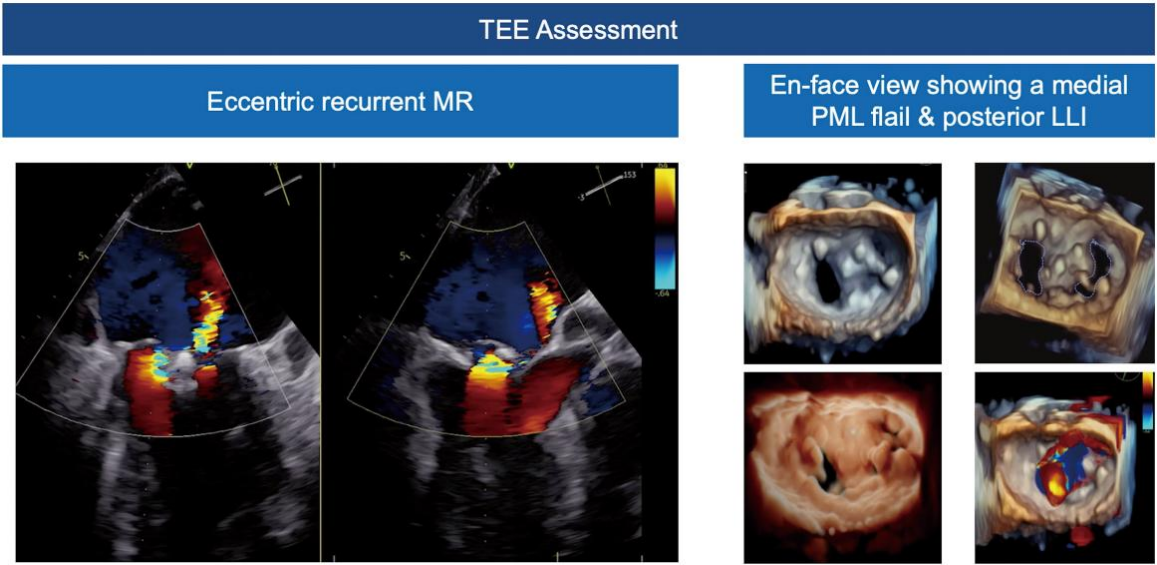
Baseline echocardiography showing recurrent mitral regurgitation after mitral transcatheter edge-to-edge repair with two PASCAL. LLI, loss of leaflet insertion; MR, mitral regurgitation; M-TEER, mitral transcatheter edge-to-edge repair; PML, posterior mitral leaflet; TEE, transesophageal echocardiography.
